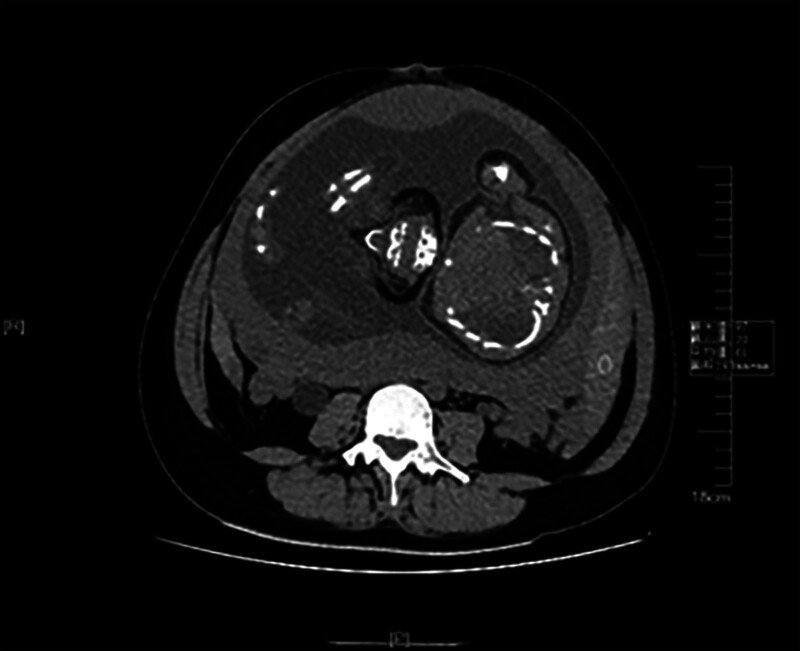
Computed tomography demonstrates pelvic and abdominal effusion.
